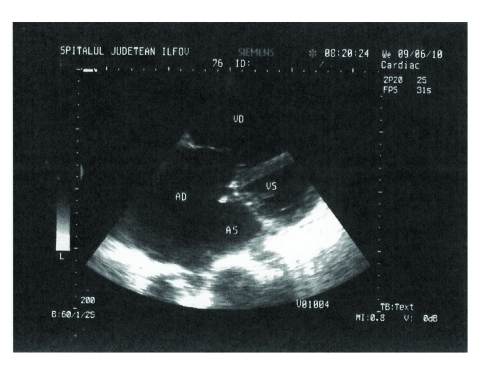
2D Transthoracic echocardiography. The right atrium dilated in apical 4 chamber–view. AD=right atrium, AS=left atrium, VS=left ventricle, VD=right ventricle.
